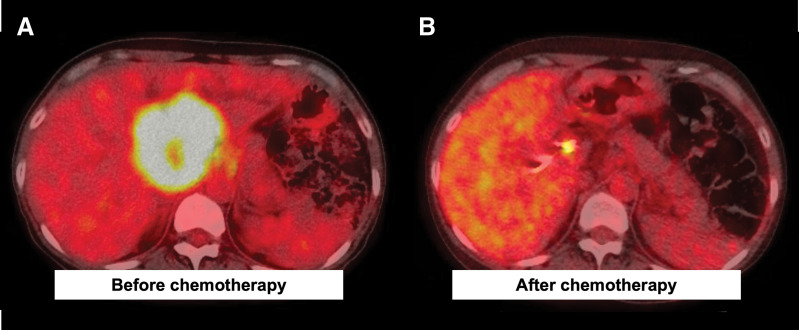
FDG-PET/CT during chemotherapy. (A) Before starting chemotherapy, FDG-PET/CT showed intense uptake (SUVmax = 12.6) in the liver. (B) After 11 cycles of GCS chemotherapy and 1 cycle of pembrolizumab therapy, FDG-PET/CT showed a remarkable reduction in both the intensity and extent of FDG uptake. The residual FDG uptake was presumed to represent reactive or inflammatory changes rather than residual tumor activity. FDG, fluorodeoxyglucose; GCS, gemcitabine, cisplatin plus S-1
